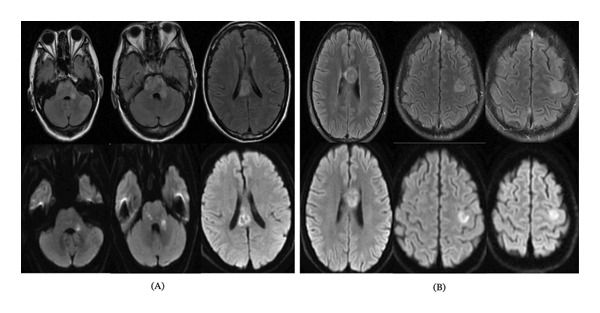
Enhanced MRI of the head of Patient 1 (A) and Patient 3 (B).
